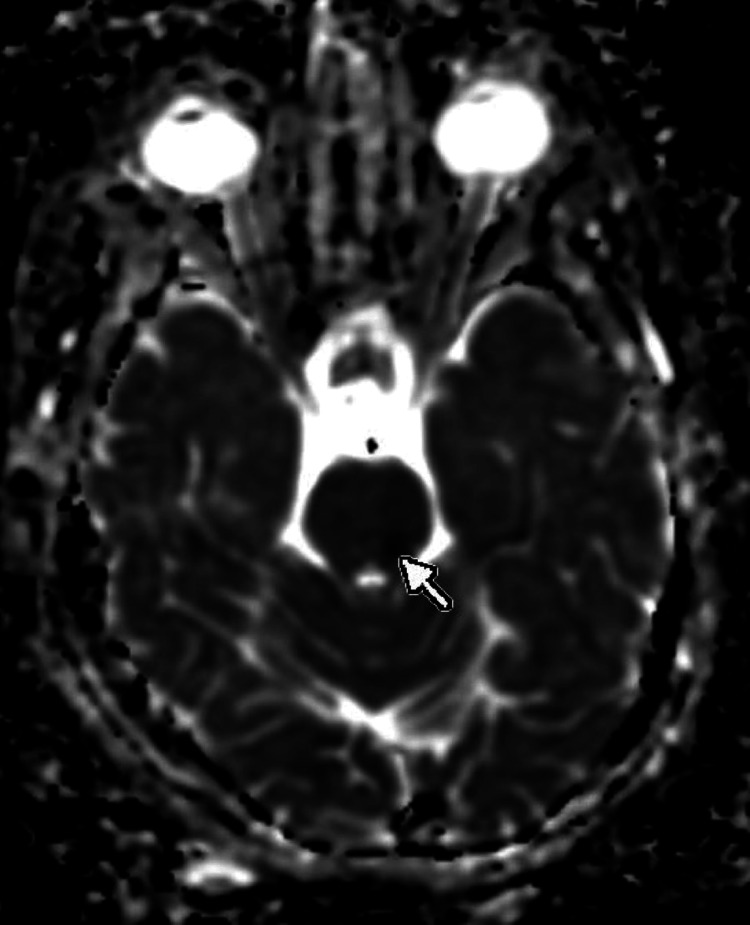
MRI of the brain showed a corresponding hypointense signal, confirming acute diffusion restriction on ADC (marked with an arrow). ADC: apparent diffusion coefficient
